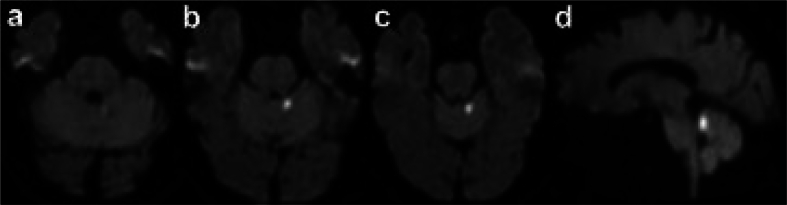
Successive axial (a-c) and sagittal (d) brain MR images. Focal ischemic lesion of the left anterior paravermis, just posterior and superior to the superior cerebellar peduncle (diffusion-weighted MRI sequences).
